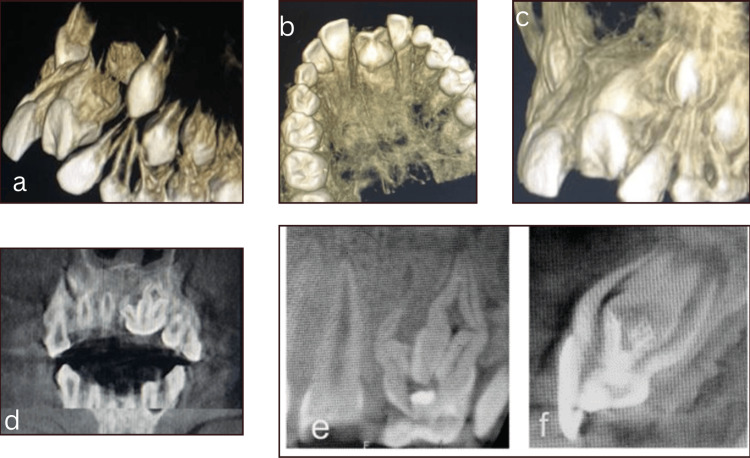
Cone beam CT images (a) Saggital 3D reconstructed view revealing the crown and root malformation in 21. (b) 3D reconstructed axial section view revealing the talon-cusp-like malformation in the incisal edge. (c) Saggital view showing crown and root dilaceration in 21. (d) Coronal sections revealing multiple roots in 21. (e) The complex morphology of the root is seen on the mesial surface. (f) The complex morphology of the root surface on the distal surface.
